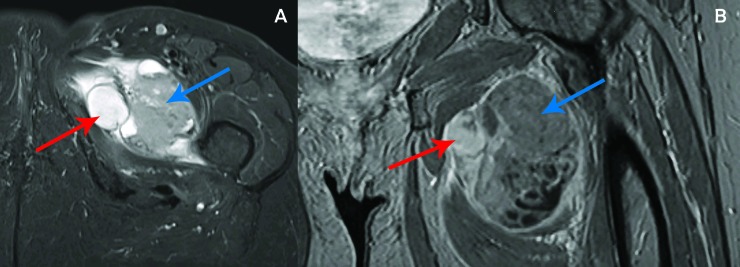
Pre-treatment magnetic resonance (MR) imaging. Axial T1 turbo inversion recovery magnitude (TIRM) (A) and coronal T1 volumetric interpolated breath-hold examination (VIBE) (B) MRI of the left upper thigh mass measuring  6.4 cm anteroposterior x 7.5 cm transverse x 7.1 cm craniocaudal shown in the axial (A) and coronal (B) plane. Note the small regions of cystic necrosis (red arrow) and surrounding soft tissue component (blue arrow).
